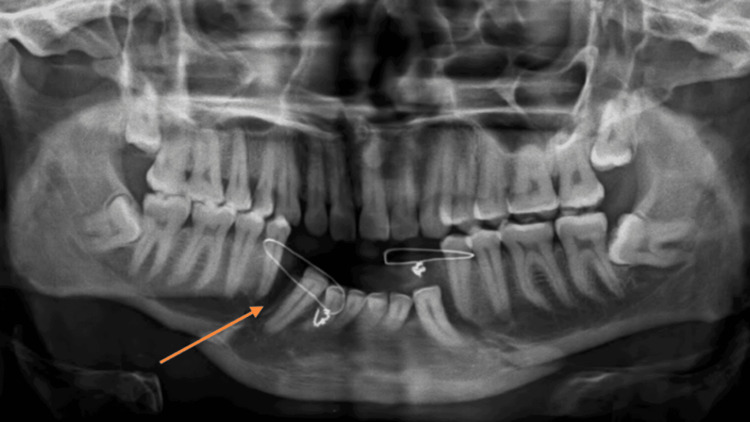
Pre-operative orthopantomogram The orange arrow indicates a bilateral para-symphysis fracture and a bilateral zygomaticomaxillary complex fracture.
